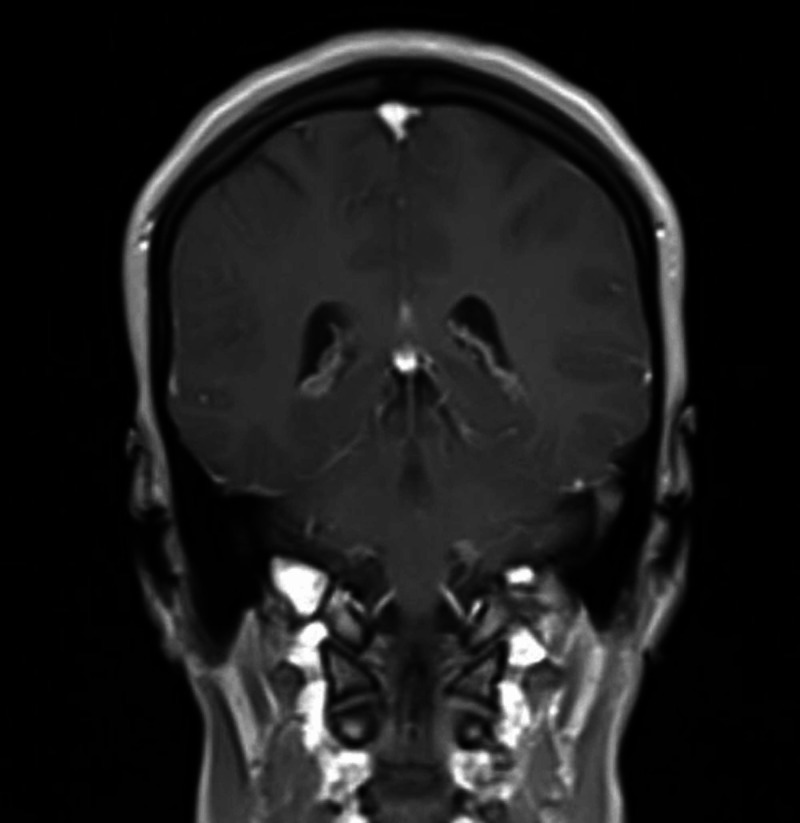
Brain MRI with and without contrast was unremarkable.
